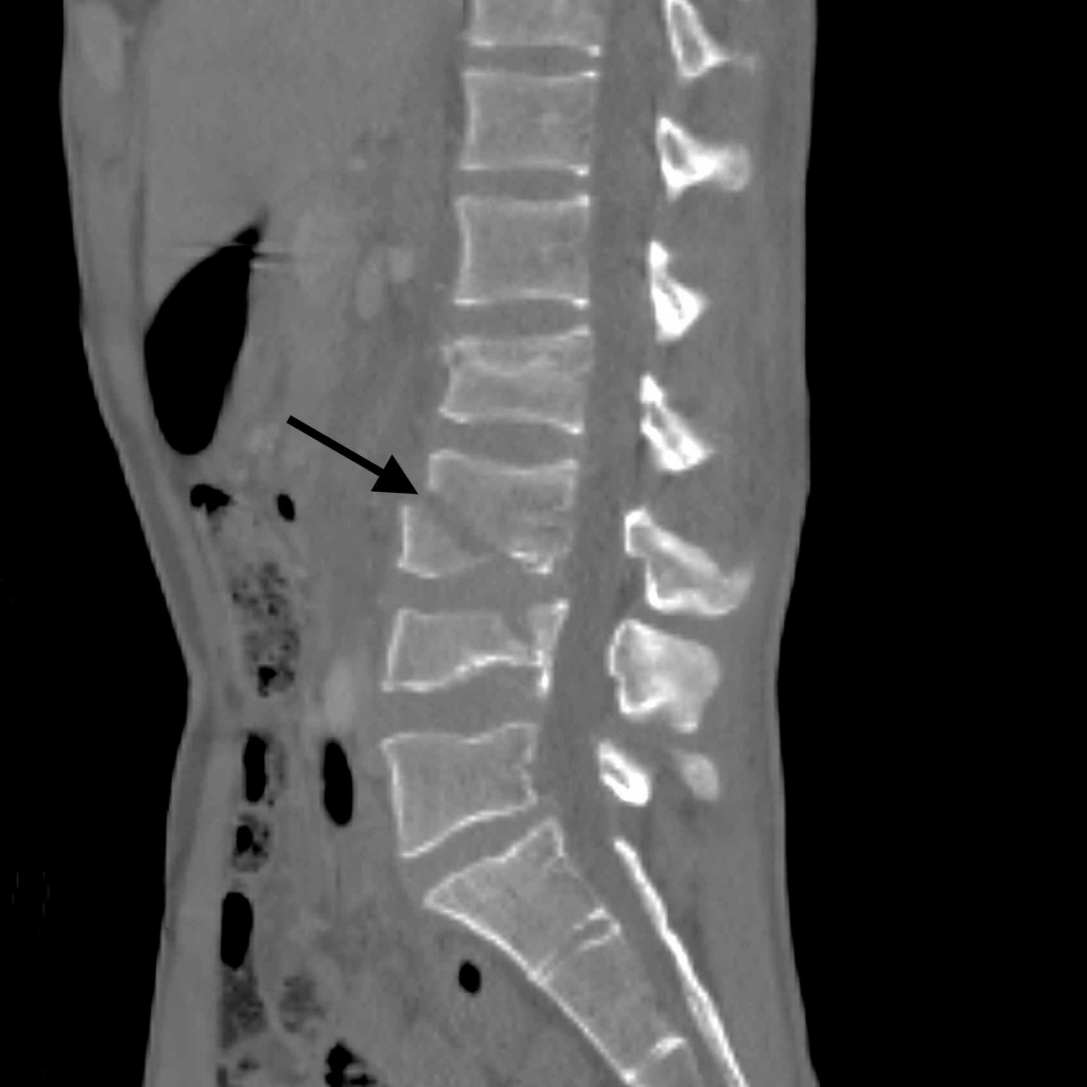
Sagittal CT demonstrating L2-4 fractures. Note the comminution of L3 and L4 resulting in a ventral defect of L3.
